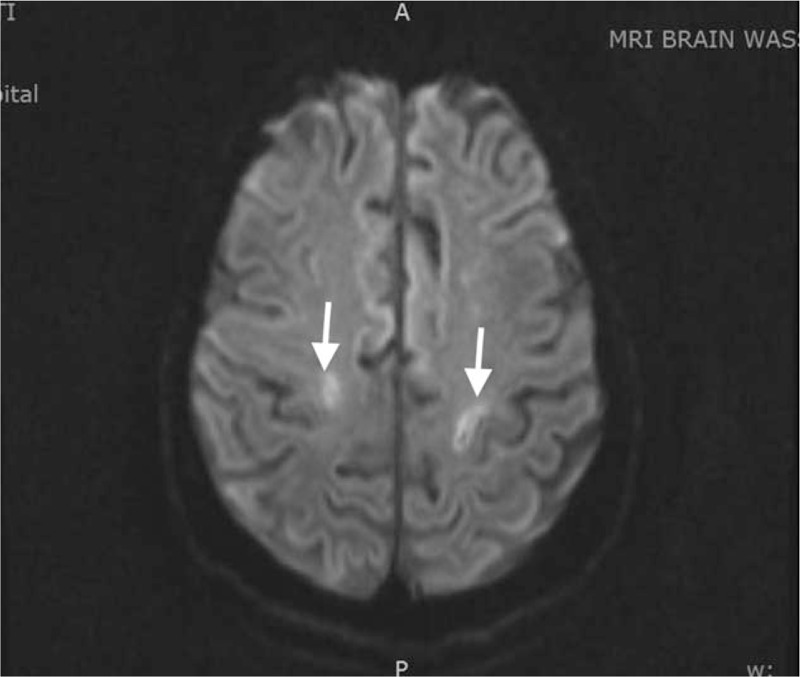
Axial MRI image after chemotherapy. The lesions mostly regressed. MRI = magnetic resonance imaging.
